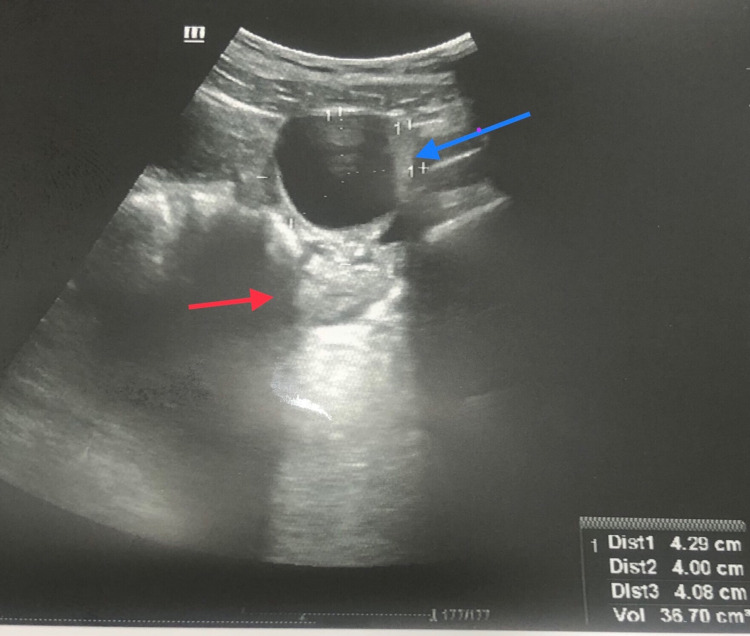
Ultrasound pelvis showing left paraovarian cyst (blue arrow) and left ovary visualized separately (red arrow).
